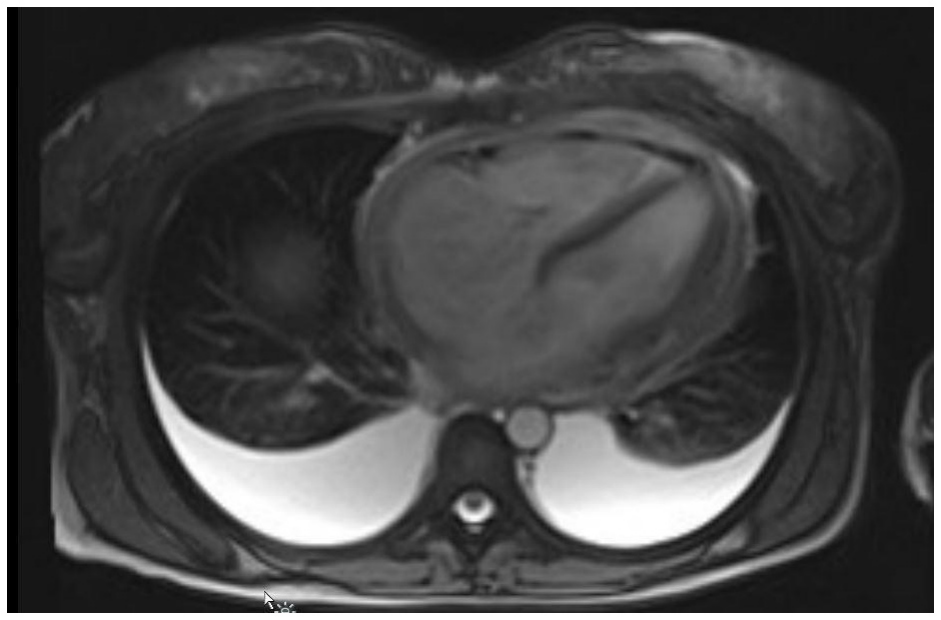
Magnetic resonance imaging shows diffuse pericardial thickening with only minimal amount of effusion.
